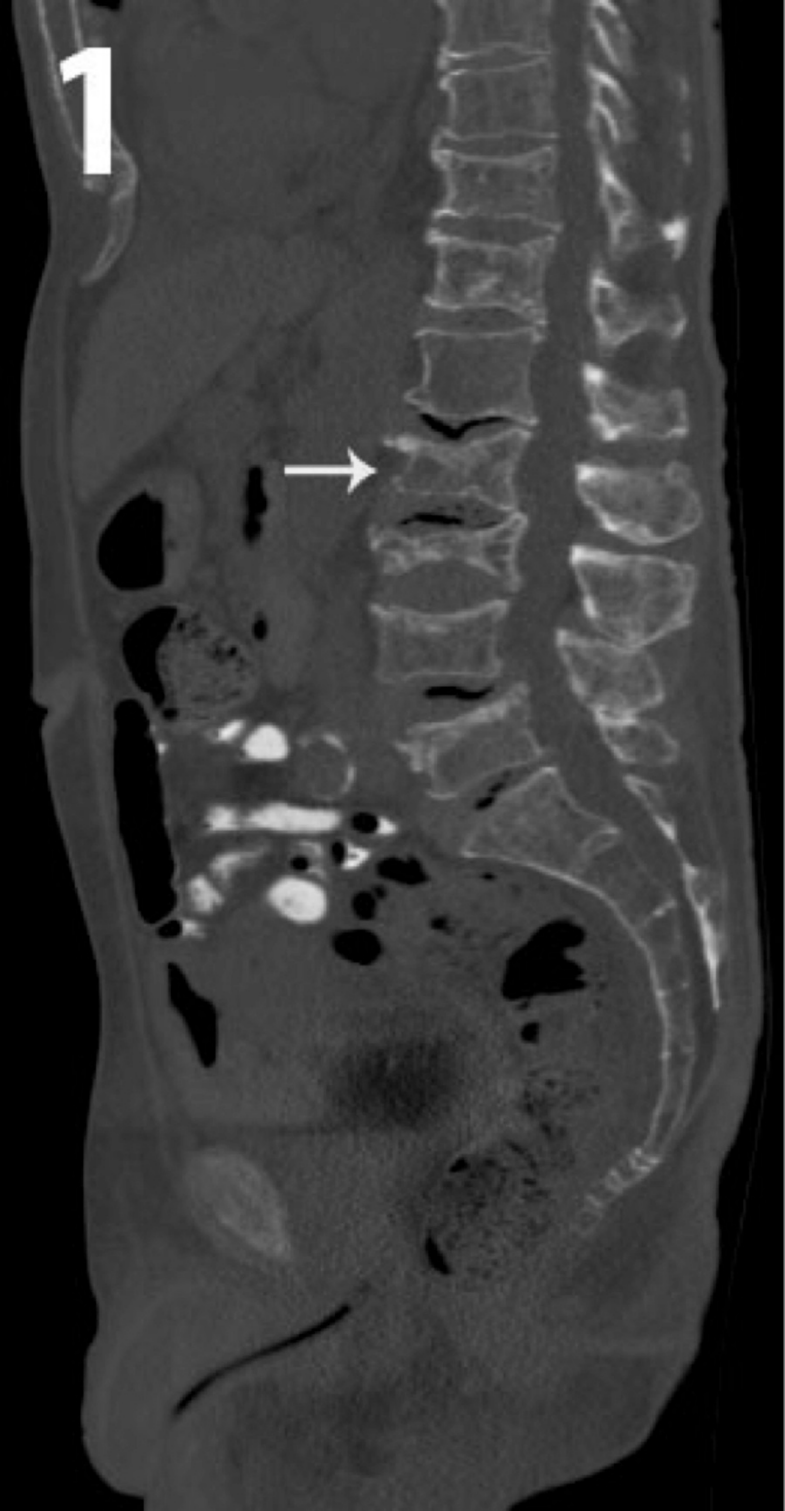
Sagittal CT image of the abdomen and pelvis using bone window levels. There is infiltration of the spine at multiple levels by multiple myeloma resulting in pathologic compression fractures (white arrow). CT- Computed Tomography
